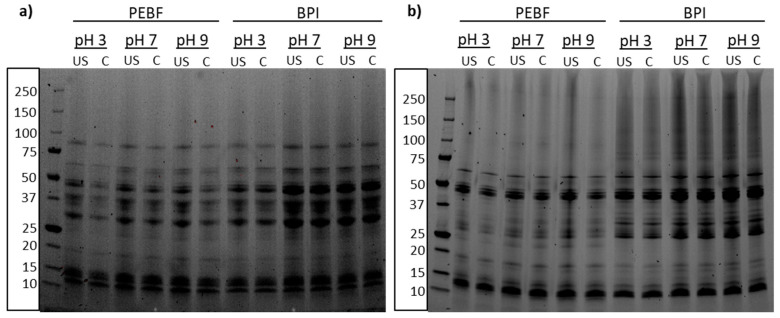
SDS-PAGE of protein-enriched barley fraction (PEBF) and barley protein isolate (BPI) at different pH-values without (C) or after ultrasound-treatment (US) analysed under (a) reducing and (b) non-reducing conditions.
